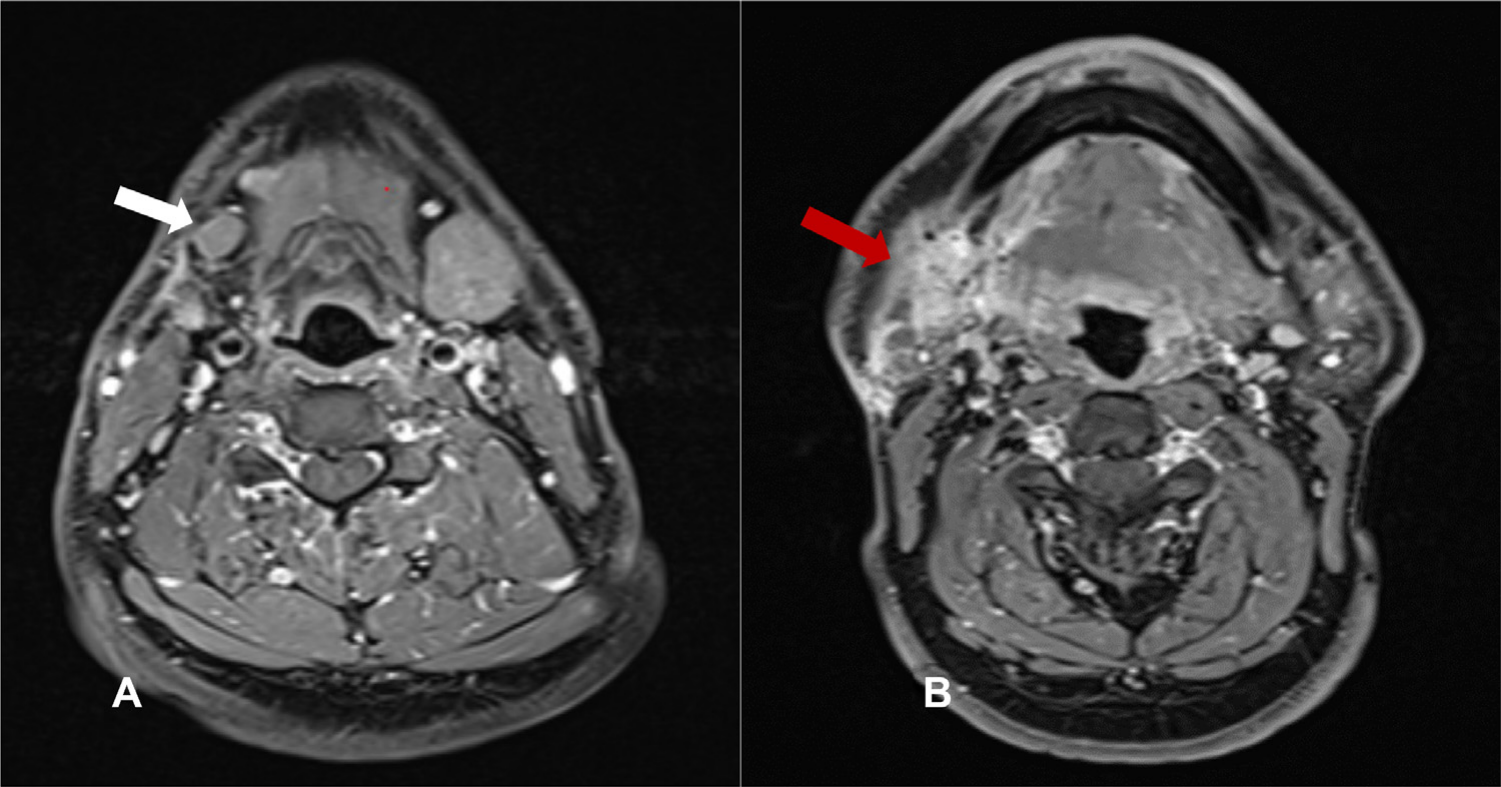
Axial T1 weighted post gadolinium MR images of the neck with fat saturation at the level of submandibular region showing an enlarged right submandibular lymph node (white arrow on Fig. 3A) with diffuse heterogeneous enhancement and fat stranding (red arrow on Fig. 3B) in the right submandibular region, suggesting granulation tissue and scar formation.
